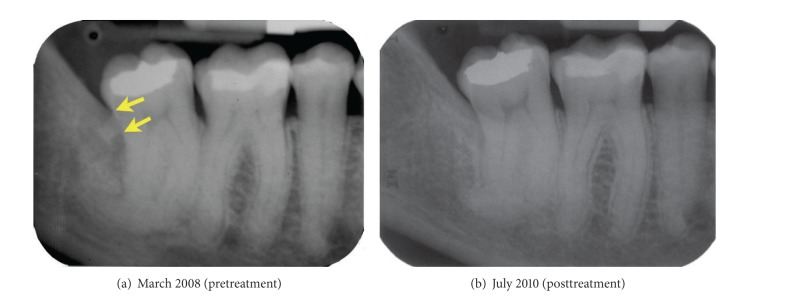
Periapical radiographs of the mandibular right posterior teeth. Radiograph (a) was obtained one month prior to the patient's initial consultation appointment. Arrows indicate the apical and occlusal borders of the calculus deposit. Radiograph (b) was obtained two years after completion of active periodontal treatment.
